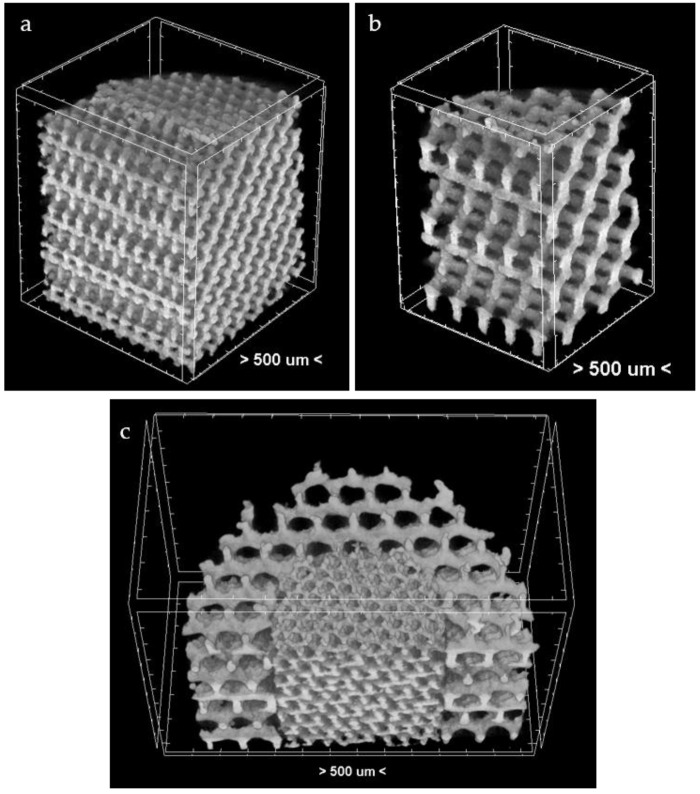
The µ-CT reconstruction of a quarter of 200 µm (a); 500 µm (b); and a half of 200 + 500 µm (c) HF/HNO_3_ polished scaffold.
